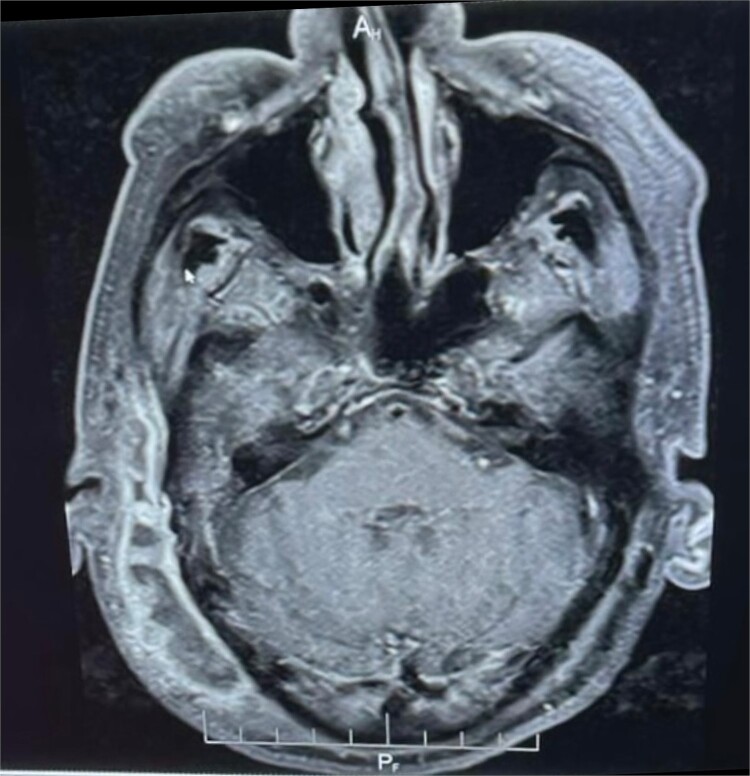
Enhanced MRI: Muitiloculated subcutaneous collection at the right temporoparietal region with marginal contrast enhancement, measuring ~0 cm x 2 cm. Marginally enhanced fluid collection on the right medial aspect of the nasopharynx.
